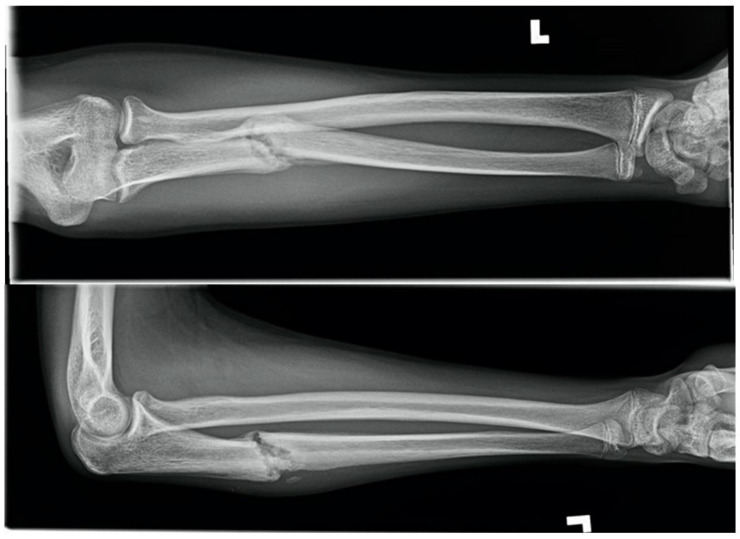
Eleven-week forearm radiographs demonstrating no union after Monteggia fracture-dislocation in a pediatric EC user.
